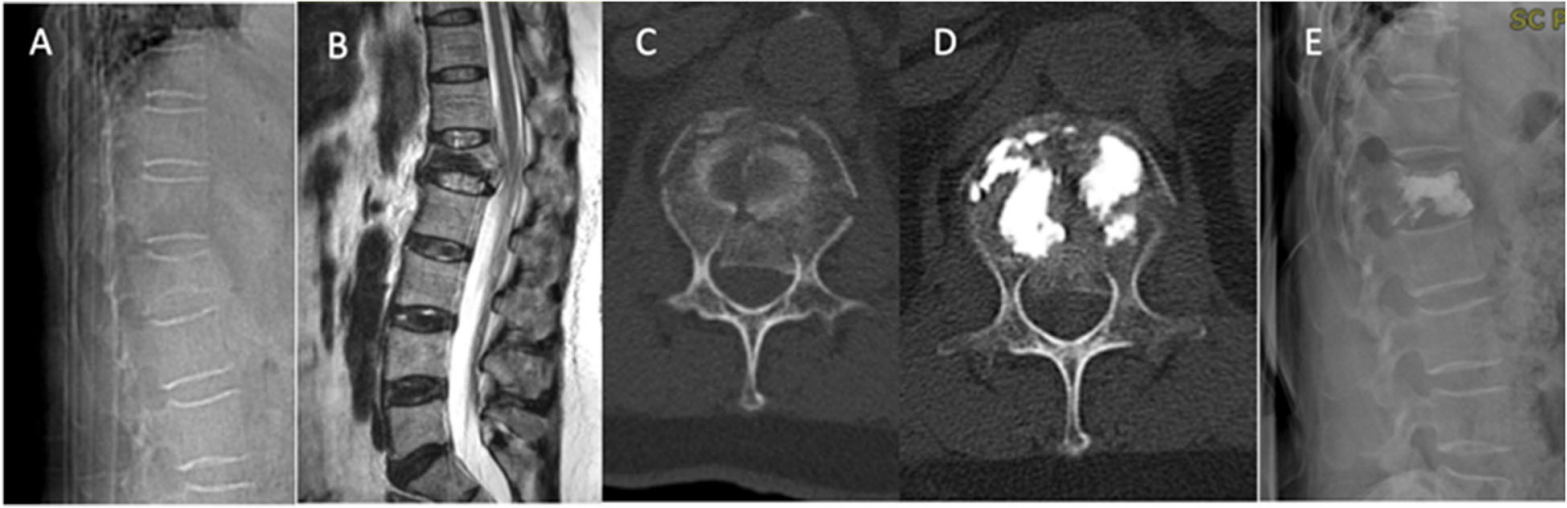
Images of patient #1. a: Preoperative X-ray radiograph of L1 fractures at the standing position; b: preoperative weighted MRI image of T2, c: axial CT image of L1; d: postoperative CT image shows the fracture area filled with cement. e: Postoperative X-ray radiographs, no cement leakage into the spinal canal
